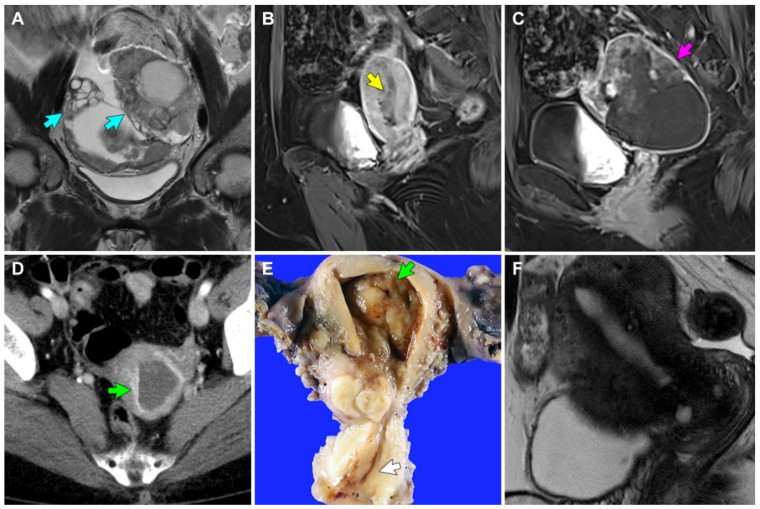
MRI, CT, and gross findings. (A) Case 1: T2-weighted coronal MR image reveals solid and cystic bilateral ovarian masses (blue arrows). (B,C) Case 2: T1-weighted Dixon sagittal MR images reveal (B) 3.4 cm endometrial mass (yellow arrow) and (C) 8.6 cm solid and cystic left ovarian mass (purple arrow). (D) Case 3: Contrast-enhanced CT image reveals a 1 cm endometrial mass (green arrow) and hematometra. (E) Case 3: An irregularly elevated mass is noted in the endometrium. The endometrial cavity is distended with blood. The endocervix (white arrow) appears unremarkable. (F) Case 4: T2-weighted sagittal MR image reveals no identifiable lesion in the endometrium.
